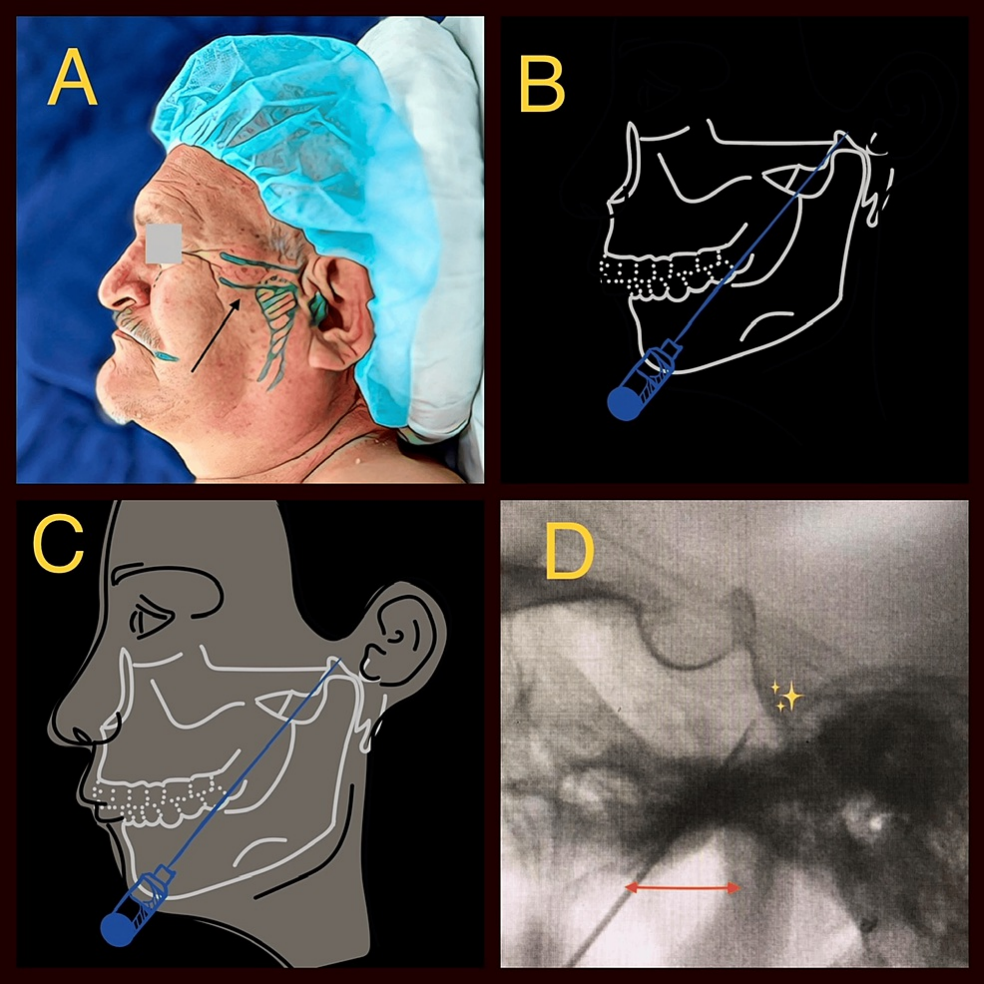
Percutaneous procedure (A) Patients were positioned supine with their heads maintained in a neutral, slightly extended orientation. The application of fluoroscopy (C-arm) in a stringent lateral position ensured precise alignment of the sphenoid planum, the floor of the sella turcica, and the ascending ramus of the lower jaw. (B and C) The puncture was executed with a 10 cm x 1 cm radiofrequency cannula, an area conspicuously devoid of Teflon. The operator's index finger was introduced into the patient's oral cavity, tracing along the upper jaw. A pivotal directive was to ensure that the needle never exceeded medial limits, safeguarding against inadvertent carotid puncture, as the foramen lacerum resides medially to the upper jaw's edge. (D) With the aid of fluoroscopy imagery, the needle was deftly guided along an imaginary projection line, passing 1 cm anterior to the temporomandibular joint (TMJ), a structural landmark impeccably discernible on strictly lateral radiographs. Image credit: This figure was created by one of the authors, Acevedo-Gonzalez JC.
